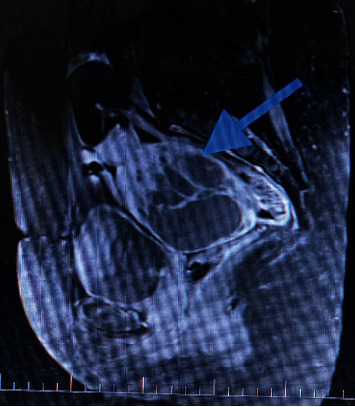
Magnetic resonance imaging, sagittal view: a solid-cystic tumour in the lesser pelvis, with thick septations. The blue arrow points to the tumour that nearly occupies the entire lesser pelvis.
